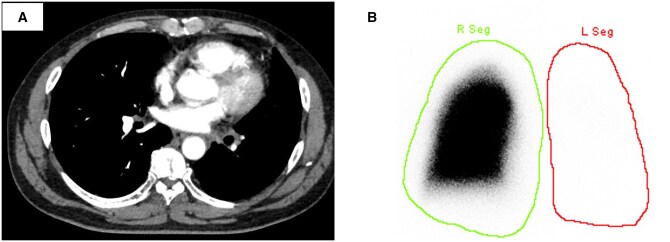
Computed tomography showed severe stenosis of the left pulmonary vein (A). Ventilation-perfusion scintigraphy showed little or no blood flow in the left lung (B).
